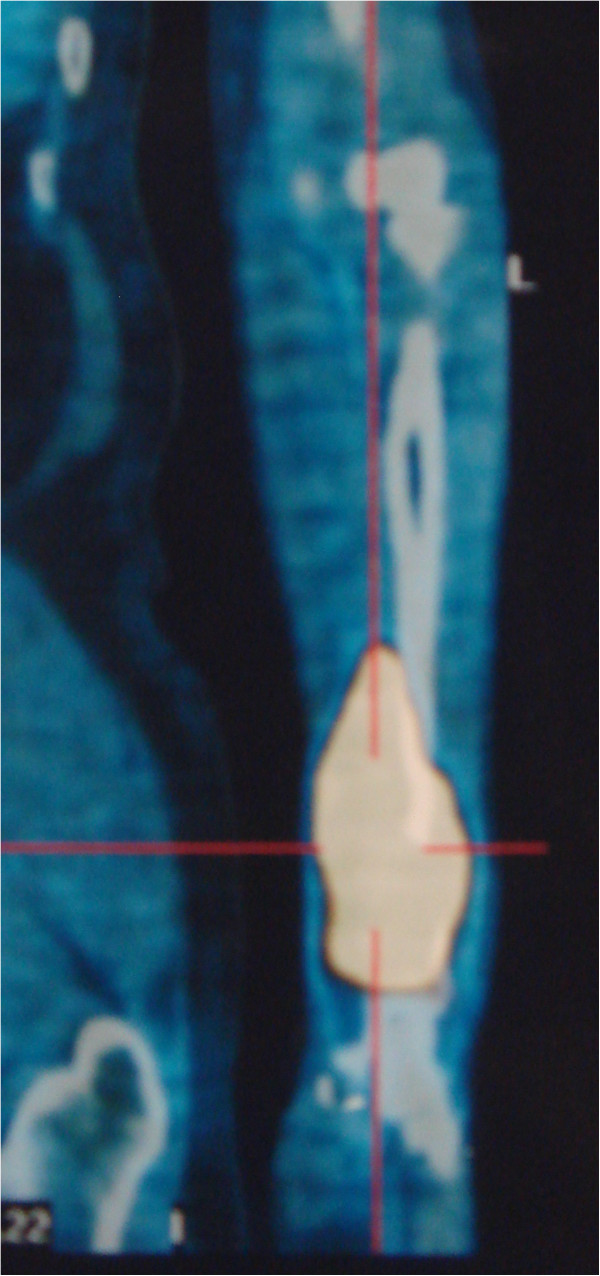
Pre-therapeutic PET/CT showed intensive FDG uptake in the distal region of the left radius. FDG, fluorodeoxyglucose; PET/CT, positron emission tomography-computed tomography.
